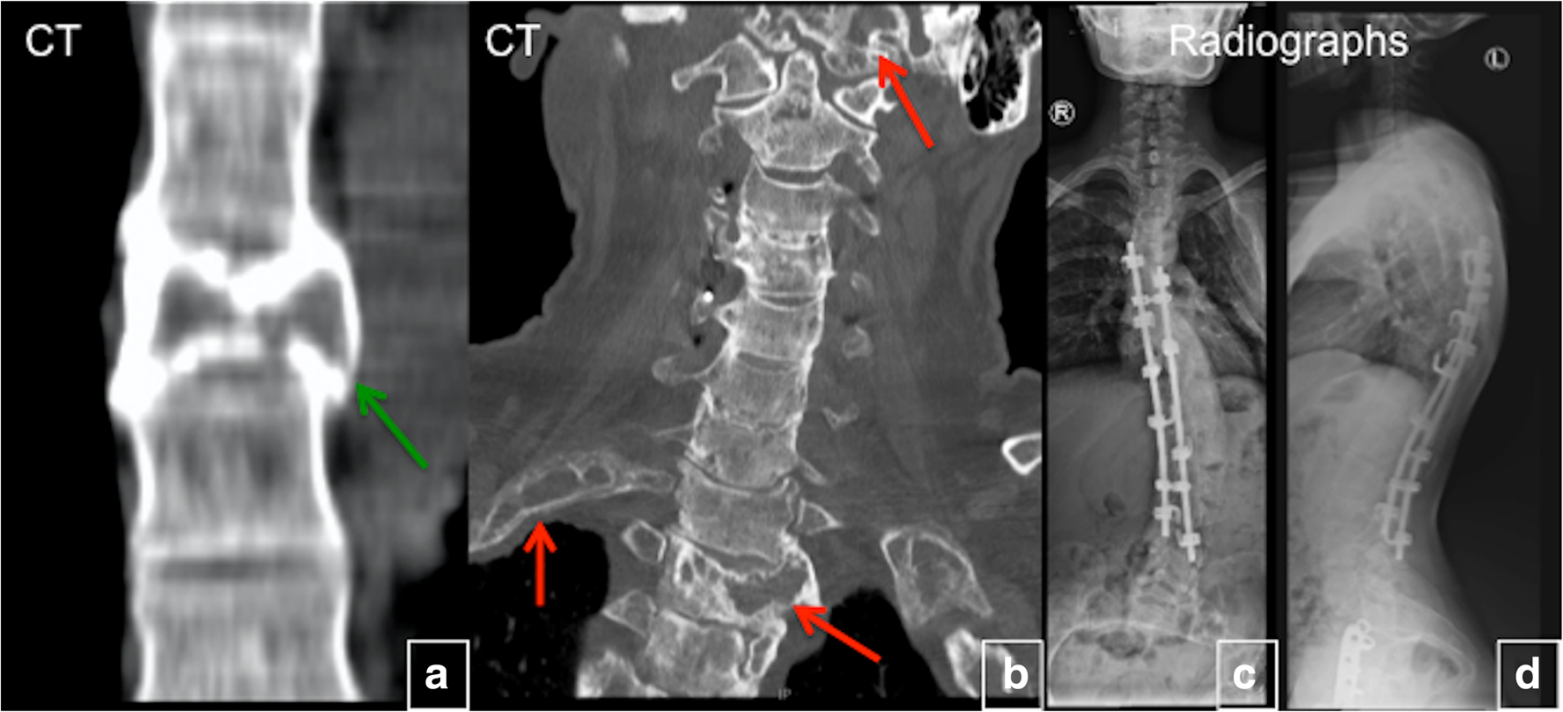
Fibrous dysplasia (FD) of the spine. a Monostotic FD of the T6 vertebral body complicated by a compression fracture (*green arrow*). b Polyostotic FD involving the spine, ribs and skull (*red arrows*). c, d Postsurgical radiographs after a spinal fusion for scoliosis in the settings of polyostotic FD
